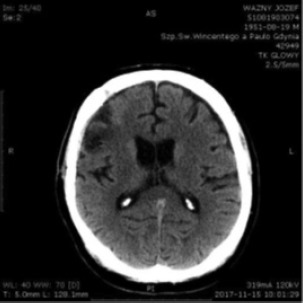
Head CT scan, 15^th^ November, 2017
